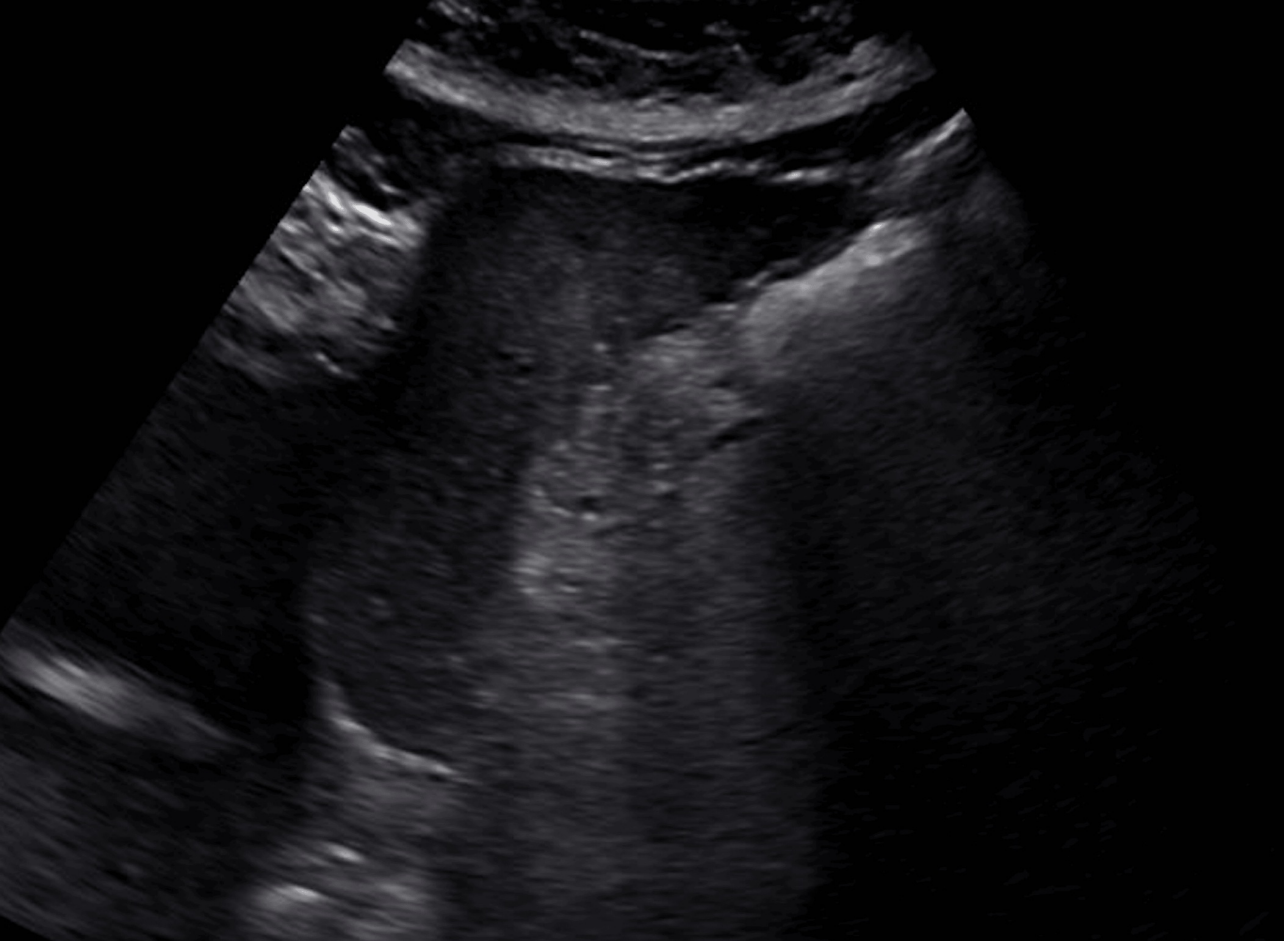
Abdominal Ultrasound Demonstrating Cirrhotic Liver Morphology Abdominal ultrasound image demonstrating heterogeneous hepatic echotexture with coarse parenchymal pattern and irregular liver surface contour, findings consistent with cirrhotic liver morphology.
